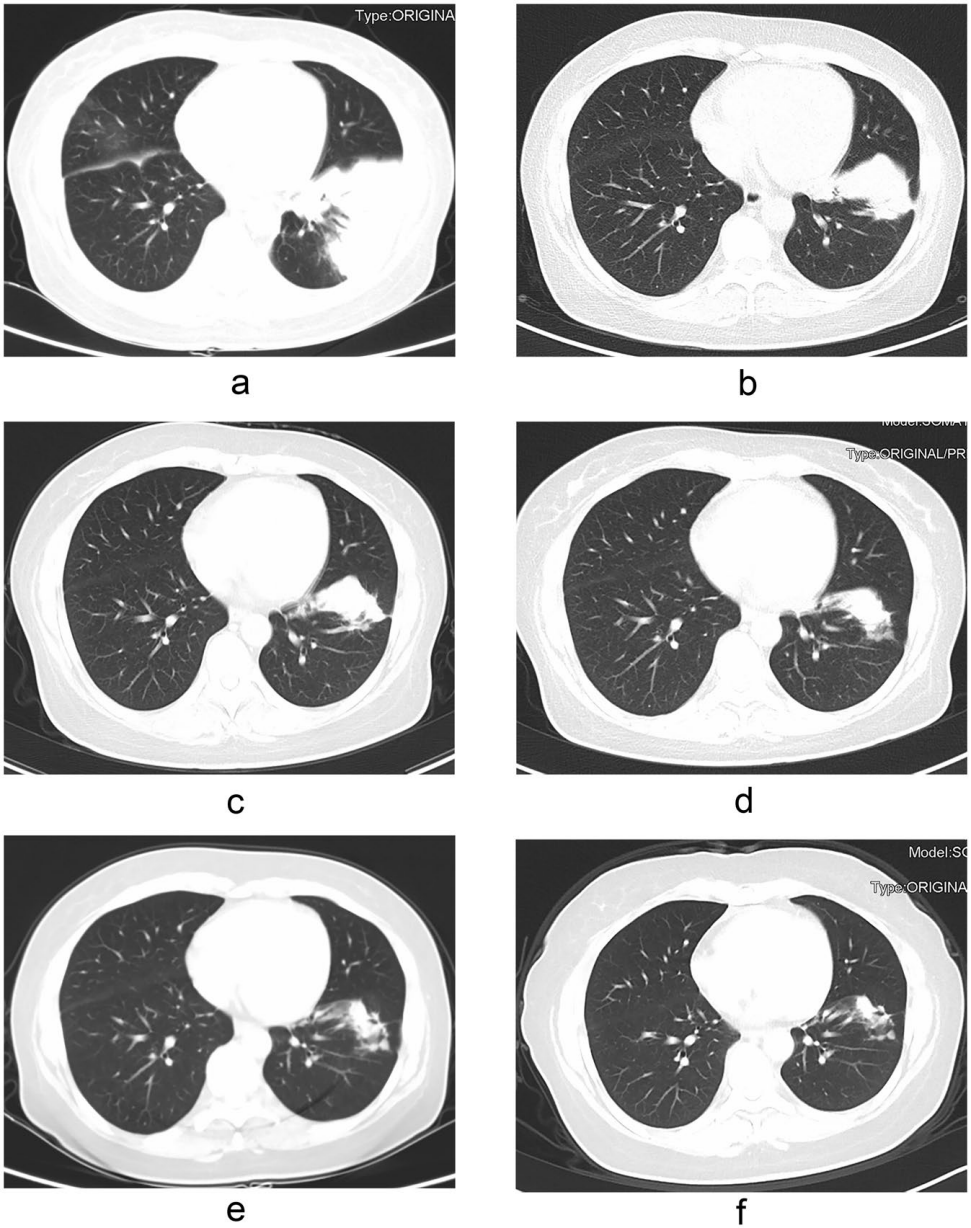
Pulmonary cryptococcosis in a 42-year-old woman with nephrotic syndrome and endometrial cancer after hysterectomy. CT imaging shows patchy consolidation in the left lower lobe of the lung (a). The lesion was significantly reduced after two months of treatment with fluconazole/voriconazole plus 5-fluorocytosine (b). The lesion continued to shrink after five months of treatment (c). The drug was discontinued after 13 months of treatment (d). The focus continued to shrink and there were still irregular soft tissue mass after 16 months (e) and 29 months (f) of drug withdrawal.
